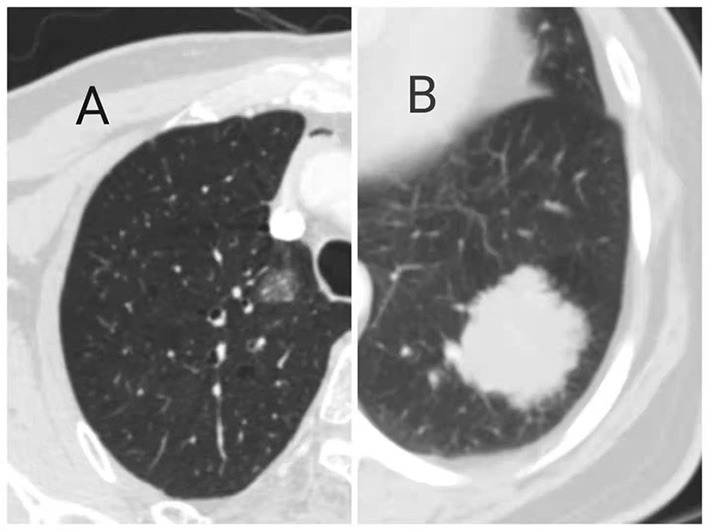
Primary pulmonary mucosa-associated lymphoid tissue (MALT) lymphoma showed ground glass (A) and solid (B) on CT imaging.
